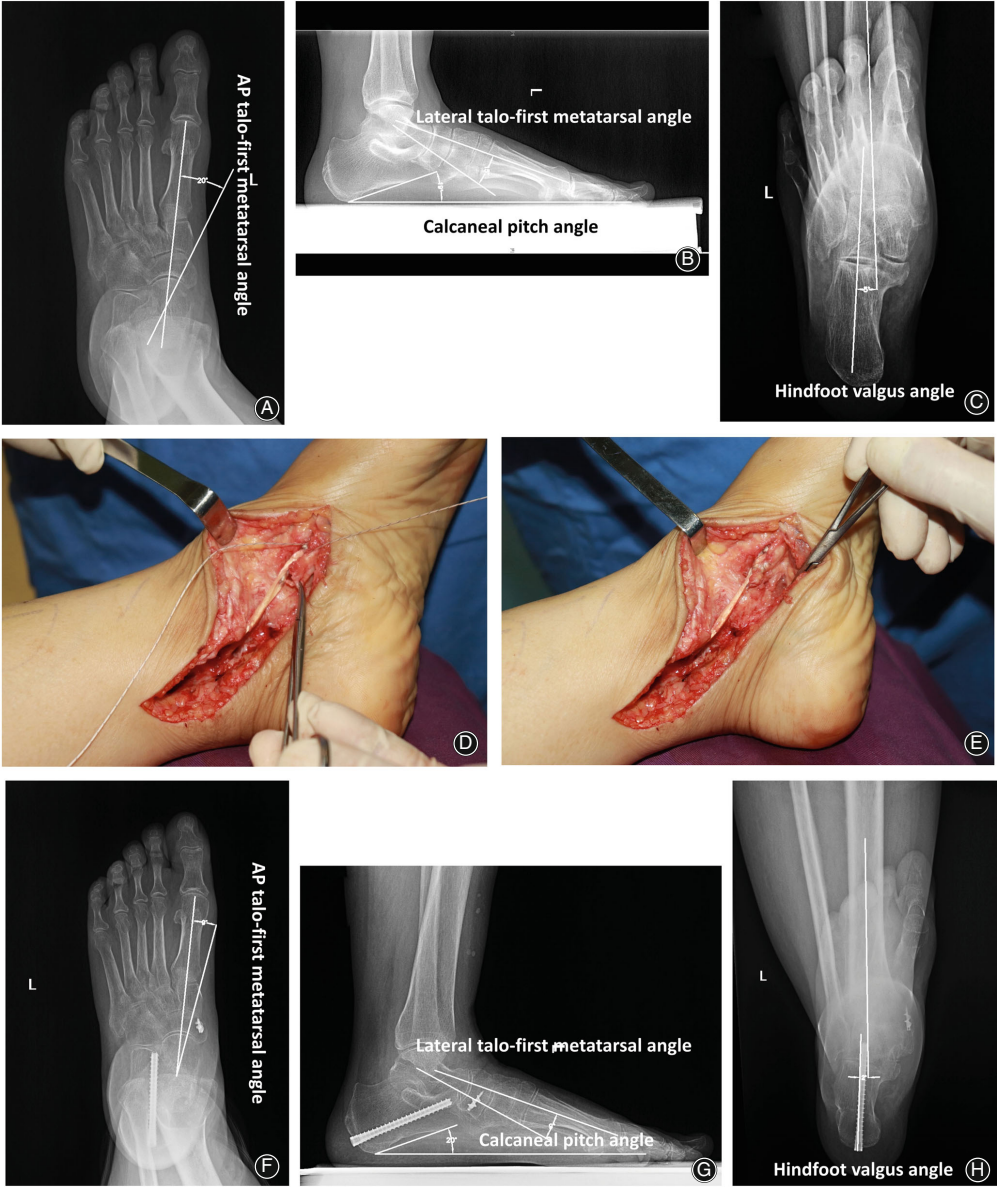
Correction of type IIA AAFD in a 55‐year‐old woman. Preoperative weight‐bearing radiographs represent anteroposterior talo‐first metatarsal angle (A), lateral talo‐first metatarsal angle, calcaneal pitch angle (B), and hindfoot valgus angle (C). After treatment with calcaneal medial displacement osteotomy and the modified PTT reconstruction (D and E), the anteroposterior talo‐first metatarsal angle (F), lateral talo‐first metatarsal angle, calcaneal pitch angle (G), and hindfoot valgus angle (H) were improved significantly on postoperative weight‐bearing radiographs
